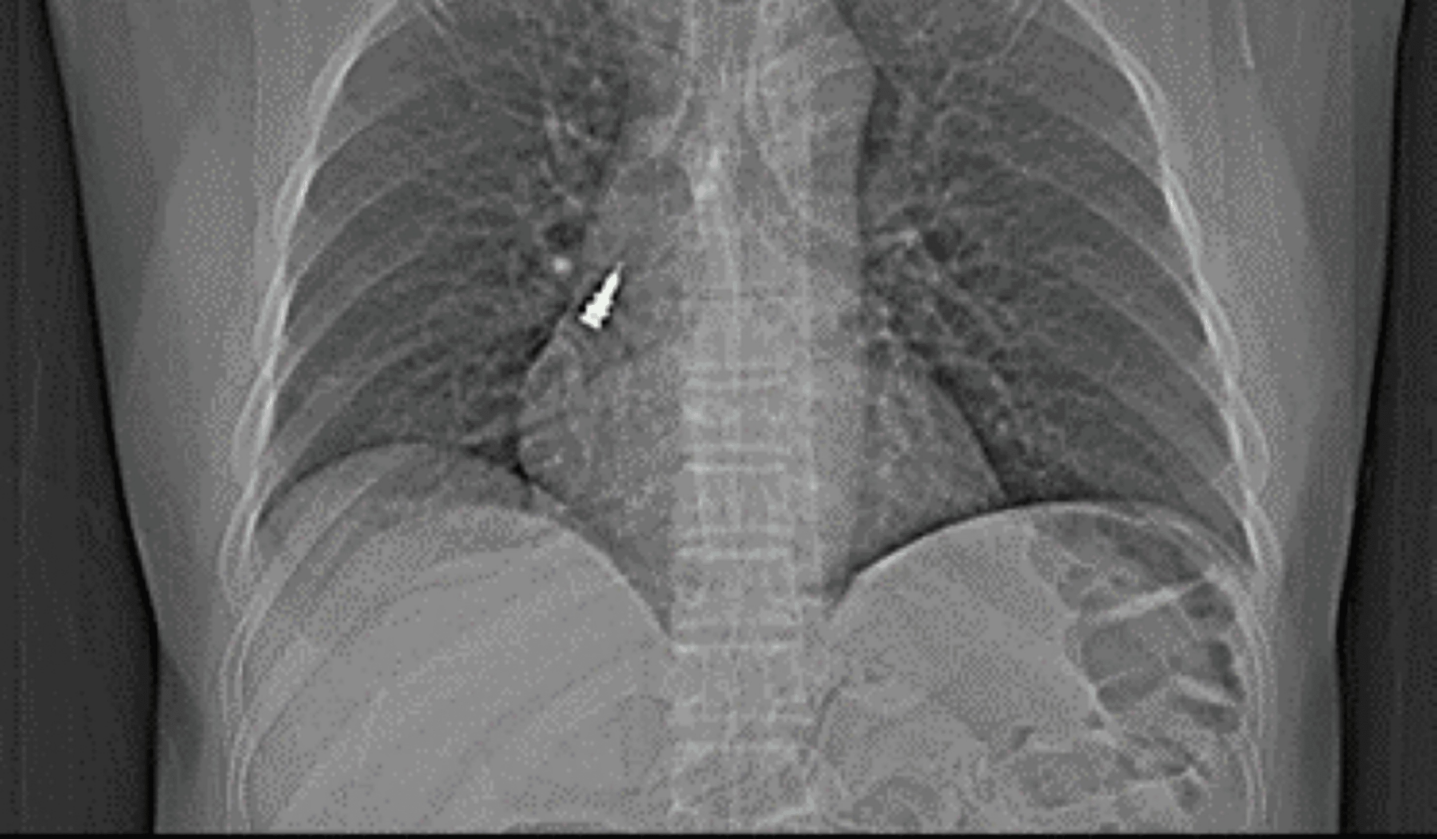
Chest X-ray confirming the presence of an implant driver in the right bronchus.
